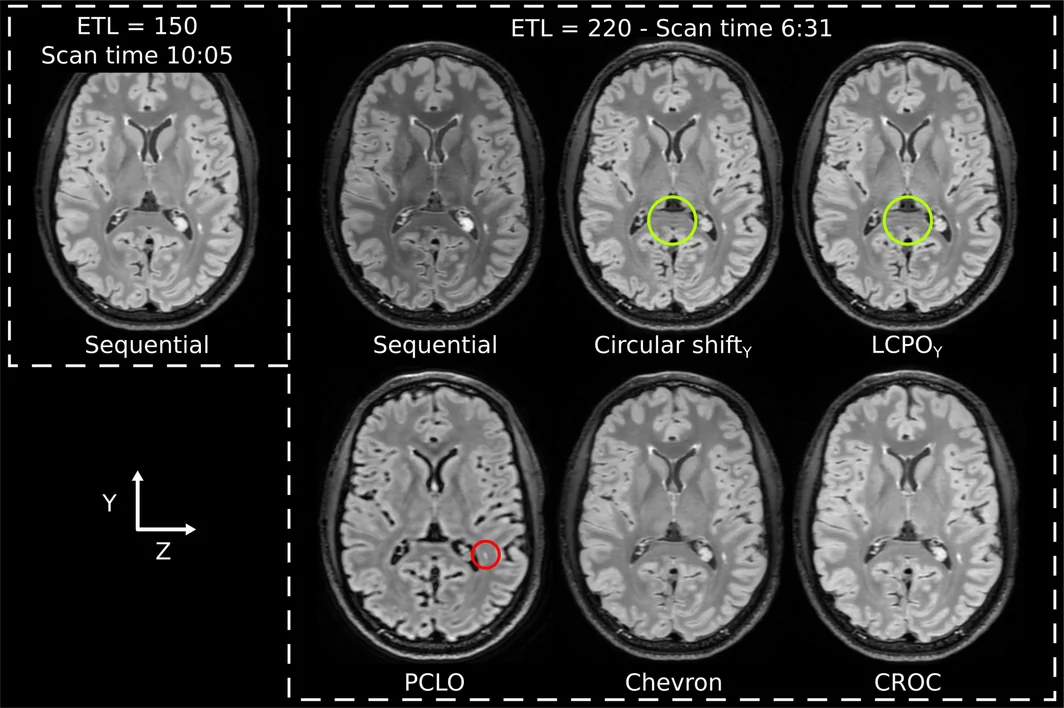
3D T2 FLAIR RARE acquired with vendor‐provided default parameters (ETL = 220, ${\text{TE}}_{\text{eff}}$ = 124 ms). An ETL of 220 yields images with an undesired ${\text{TE}}_{\text{eff}}=174$ ms. For sequential, ETL was decreased from 220 to 150 to match the desired ${\text{TE}}_{\text{eff}}$, resulting in a scan time of 10:05. The proposed view orders were acquired with a center echo chosen to match ${\text{TE}}_{\text{eff}}$ = 124 ms, with a scan time of 6:31. Green circles highlight a ringing appearance along Y. The red circles highlight a partly obfuscated white matter lesion.
